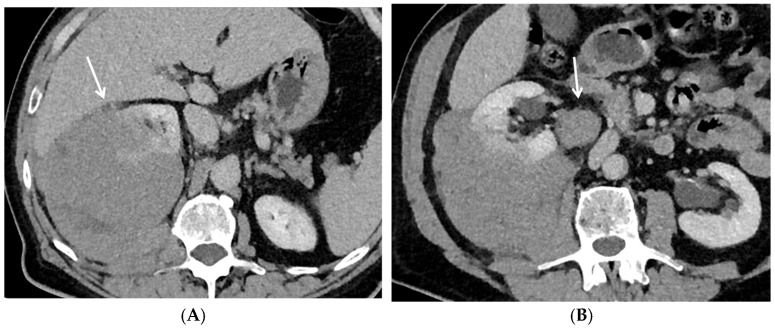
Lymphoma involving the right renal parenchyma. Contrast-enhanced nephrographic phase axial CT images (A,B) show a large infiltrative, homogeneously enhancing mass in the right kidney (arrow, A), with extension into the perirenal space (white arrow, B). Associated lymphadenopathy is noted in the renal hilum (arrow, B).
